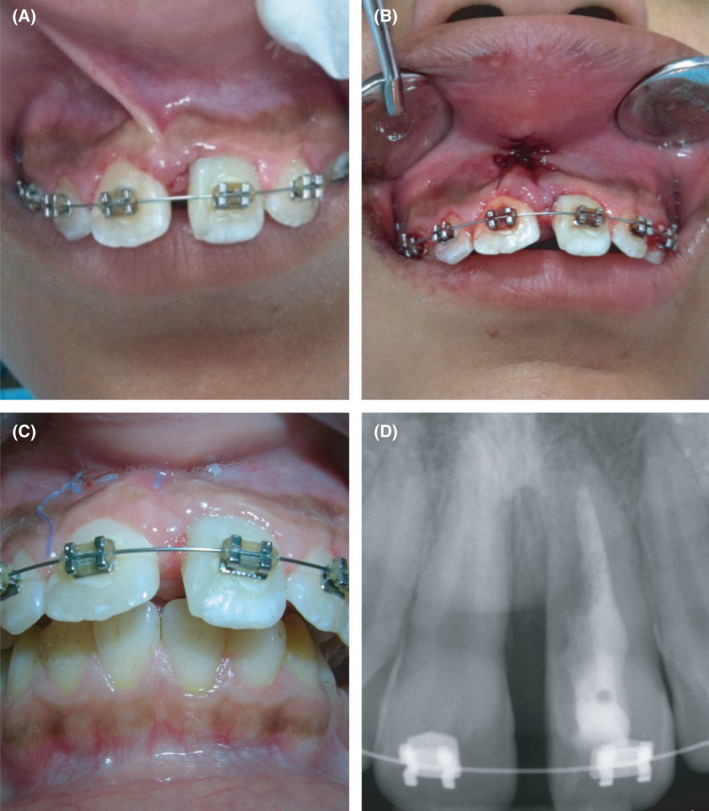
Frenectomy procedures for the patient performed 4 months after the initial surgical intervention. (A) shows high frenal attachment; (B) immediate post‐operative photo after frenectomy procedure with sutures in place; (C) Healing 2 months after frenectomy; (D) Periapical radiograph 2 months following frenectomy procedure
